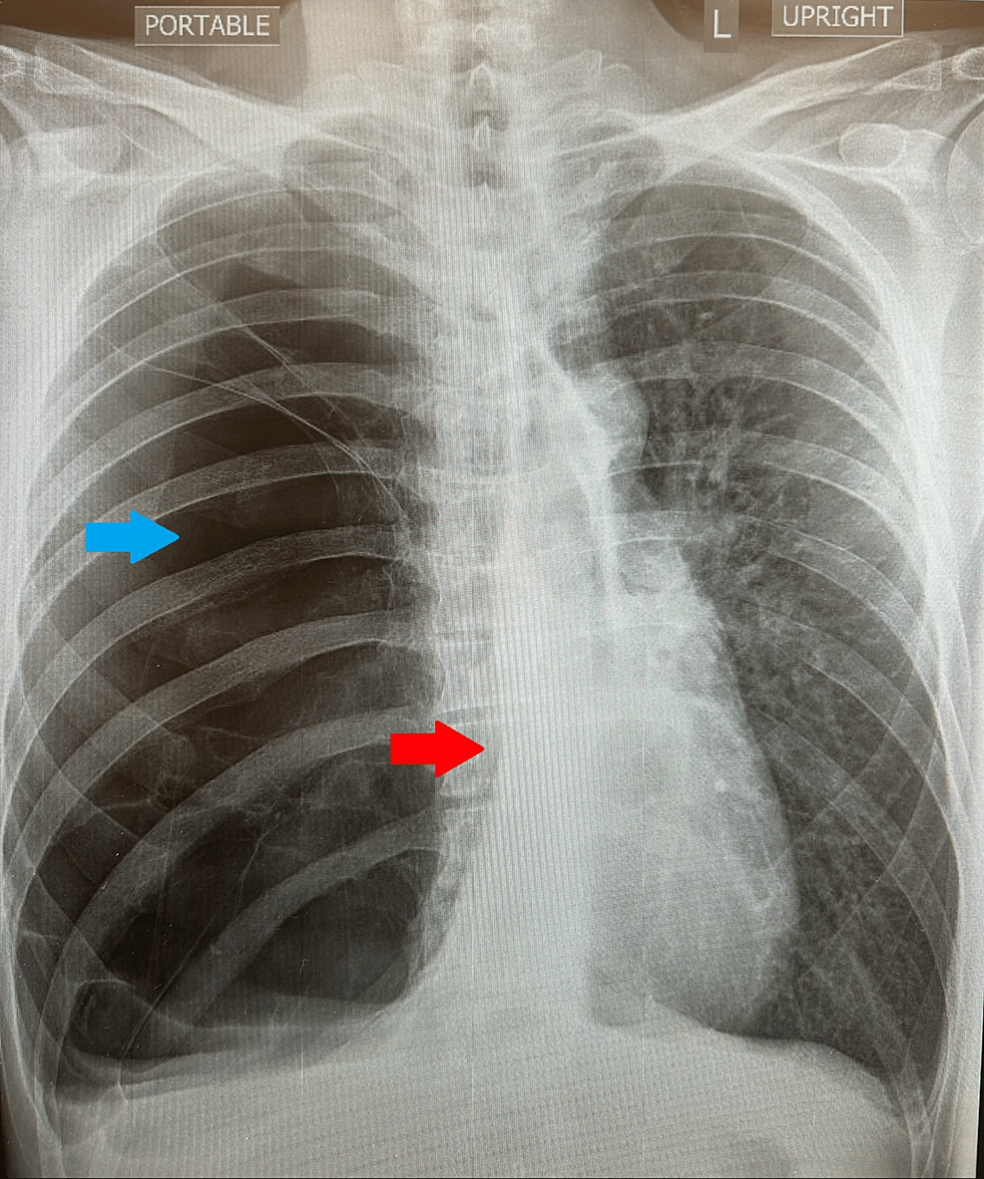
Chest X-ray of the 50-year-old male patient The plain radiograph depicts hyperlucency (blue ➨) of the right hemithorax and a subsequent mass effect causing a left mediastinal shift (red ➨).
